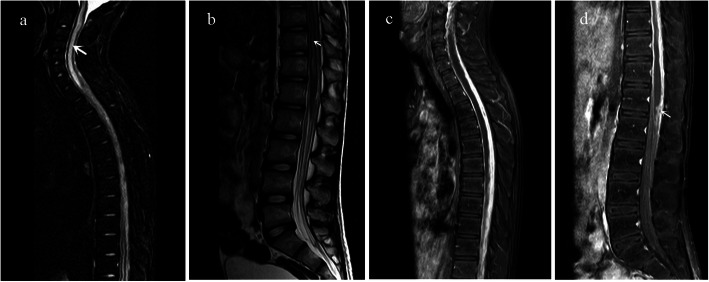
a Sagittal fat-suppressed T2-weighted MRI scan of the spine demonstrating notable dilation of the central canal of the cervical cord (Syrinx formation) from C1 to T1 (arrow). b Sagittal T2-weighted image of the spine demonstrating hyperintense signals within the lumbar cord (arrow) and conus medullaris. c Sagittal post-contrast T1-weighted image demonstrating extensive thickening and enhancement of the arachnoid, disappeared subarachnoid space, and atrophy of thoracic spinal cord. d Sagittal post-contrast T1-weighted image demonstrating nodular enhancement of conus medullaris (arrow)
